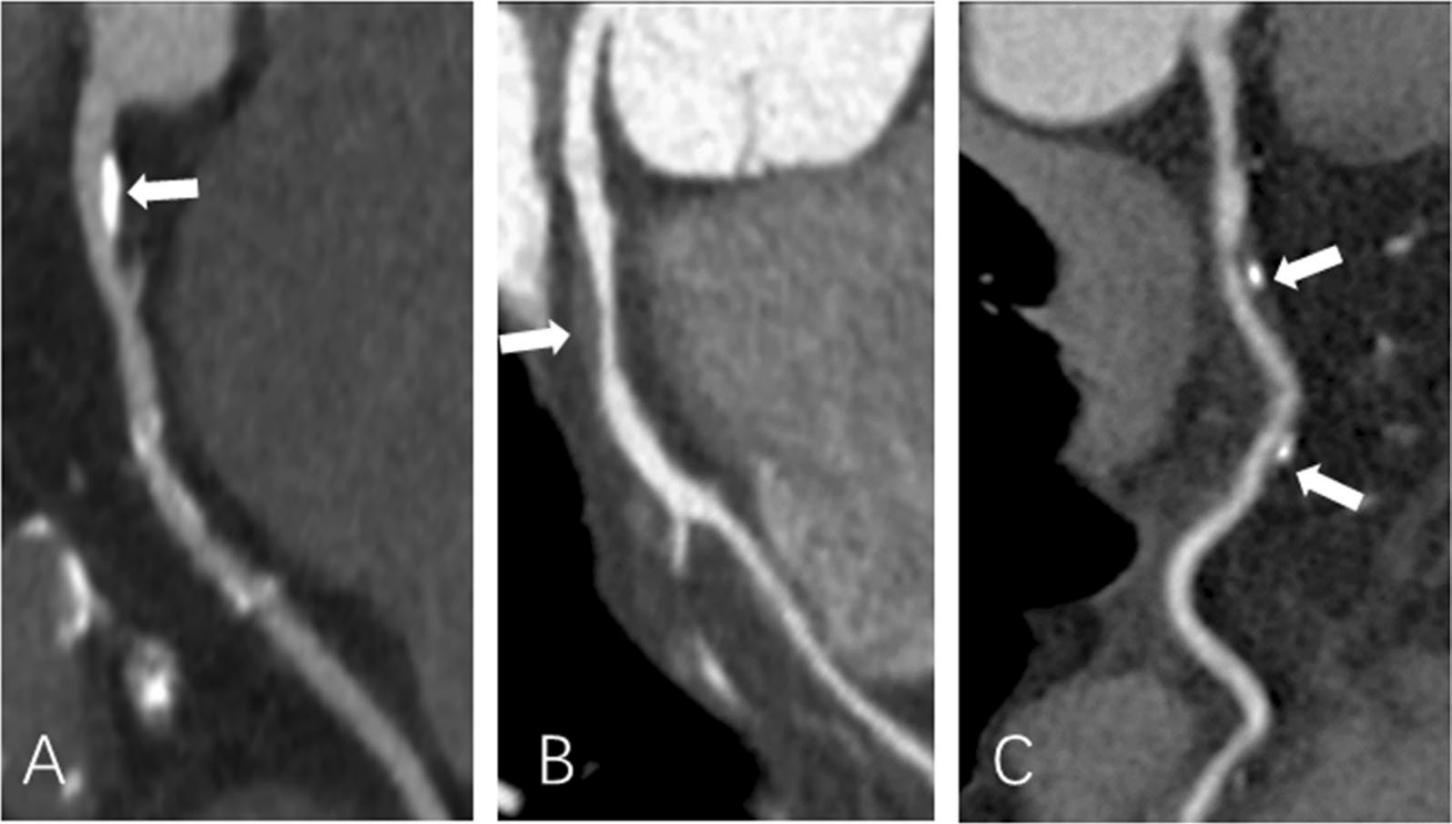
Representative coronary computed tomography angiography images of A calcified plaque, B noncalcified plaque, and C mixed plaque
